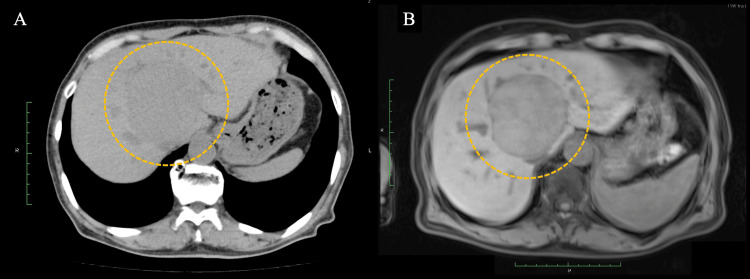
Images at diagnosis (A) Abdominal CT demonstrates a 70-mm hepatocellular carcinoma in hepatic segment 1 (S1) (dotted circle). (B) Abdominal MRI likewise showes a lesion at the same site on T1-weighted sequences. The disease was confined to a single lesion in hepatic S1 (dotted circle).
